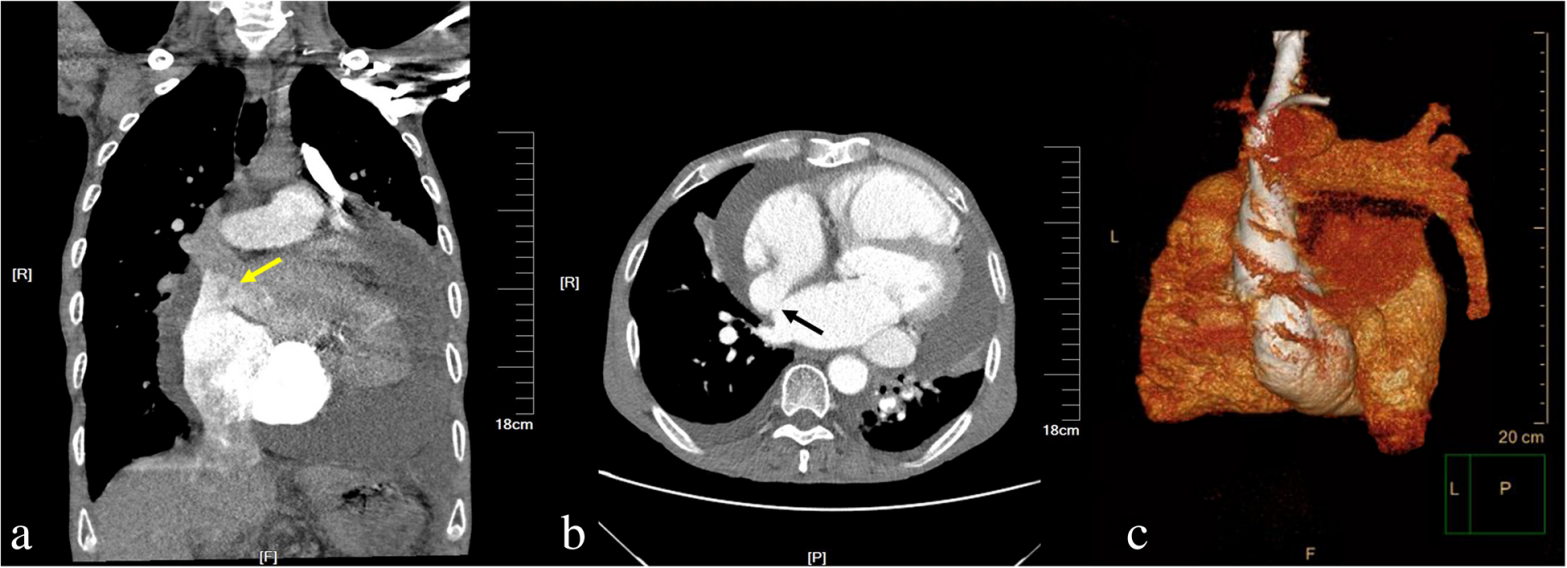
Emergency thoracic contrast enhanced computed tomography revealed cardiovascular anomalies. a Contrast agent slightly appeared in left atrium (yellow arrow) when the right heart was enhancing. b Interruption was visible between right atrium and left atrium. c A persistent left superior vena cava drained into the right atrium through the aneurysmal dilated coronary sinus
